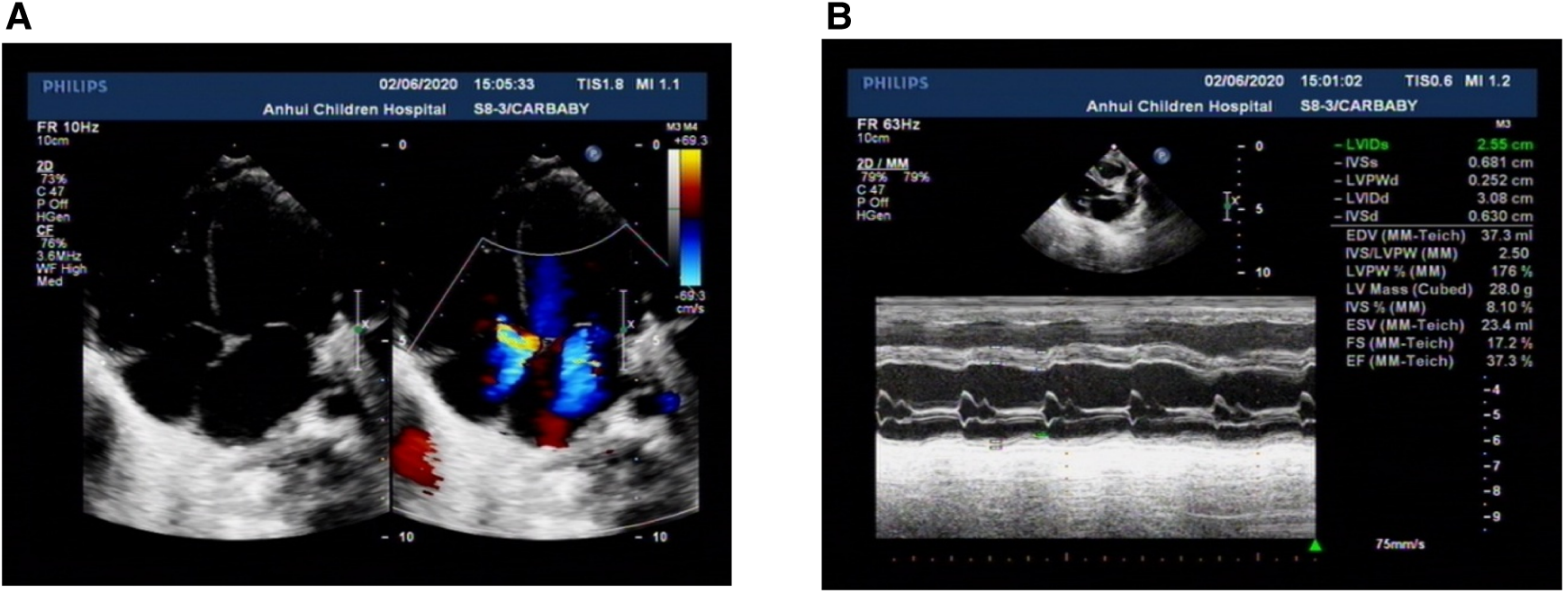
Characteristics of Doppler echocardiography. (A) All four ventricles of the heart were enlarged, and the left ventricle was prominent. (B) Left ventricular systolic dysfunction was low. All the parents were normal.
